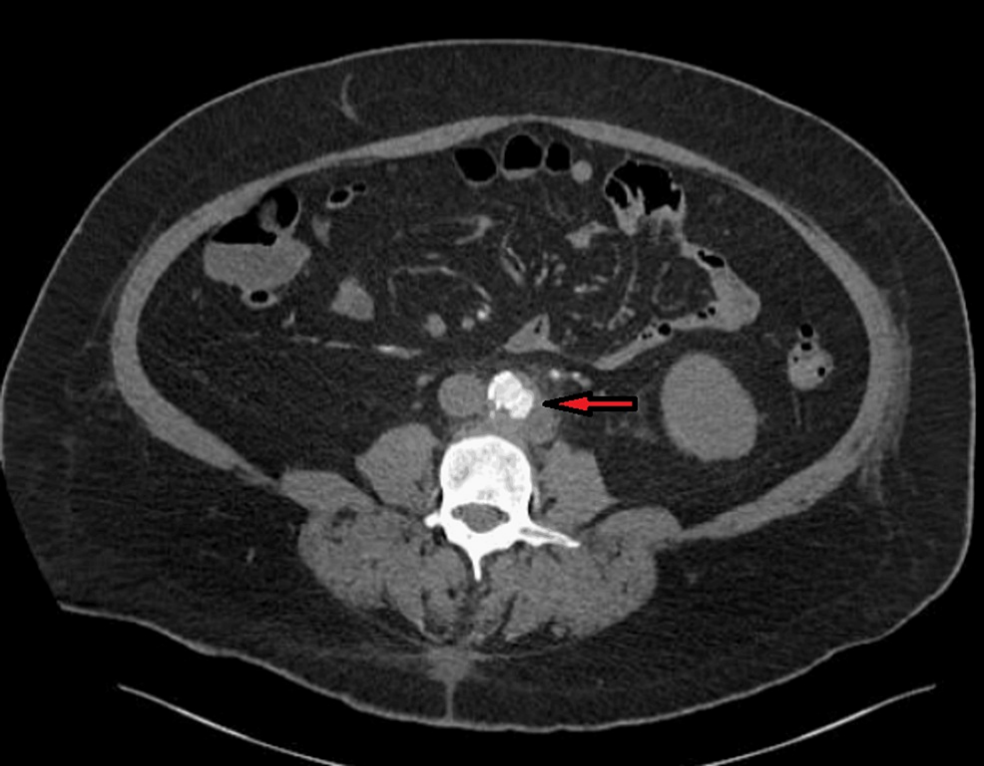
Axial view of CT angiogram of the abdomen Axial view of the CT angiogram of the abdomen demonstrating inflammatory stranding just cranial to the aortic bifurcation with an associated ulcer (arrow).
